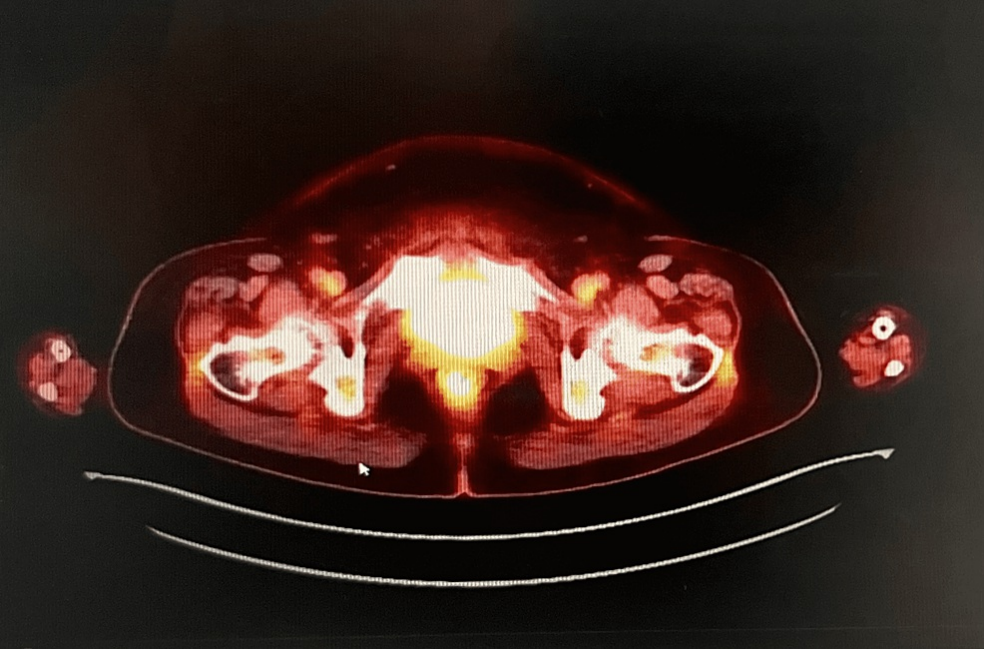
Restaging PET-CT scan at the completion of chemotherapy showing no evidence of any malignant activity in the uterus. PET-CT: positron emission tomography-computed tomography
